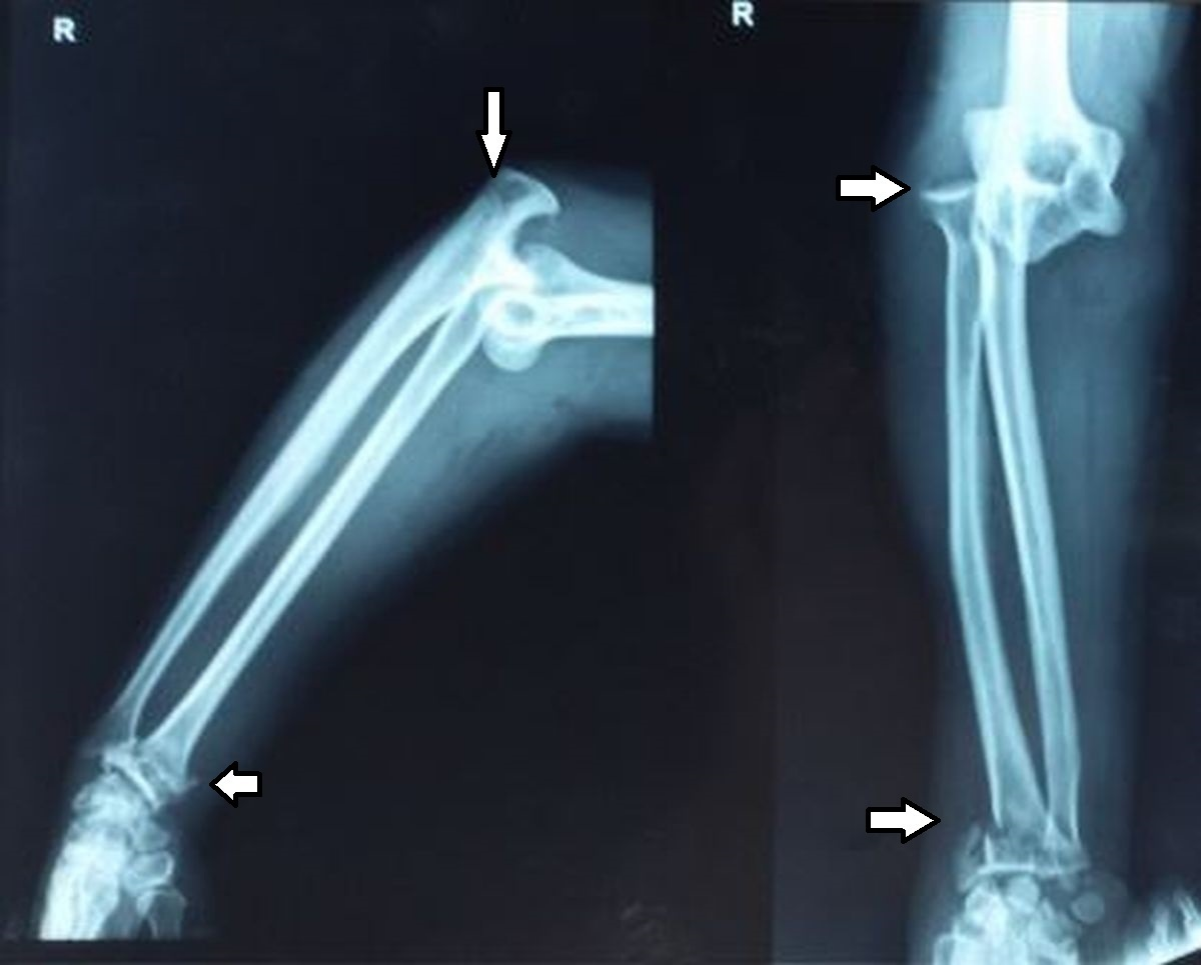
Pre-reduction plain radiograph of the right elbow and wrist, anteroposterior and lateral views Distal radius fracture with postero-lateral elbow dislocation (white arrows).
